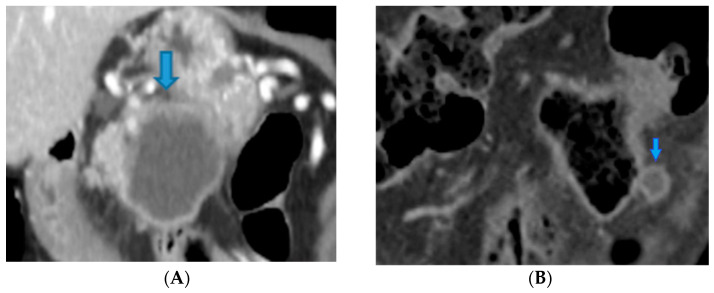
Coronal MPR CE CT PC from pancreatic cystoadenocarcinoma: Observe the primary tumour (arrow in (A)) and its deposit within the sigmoid mesocolon (arrow in (B)). Notice the hypovascular resemblance between them.
